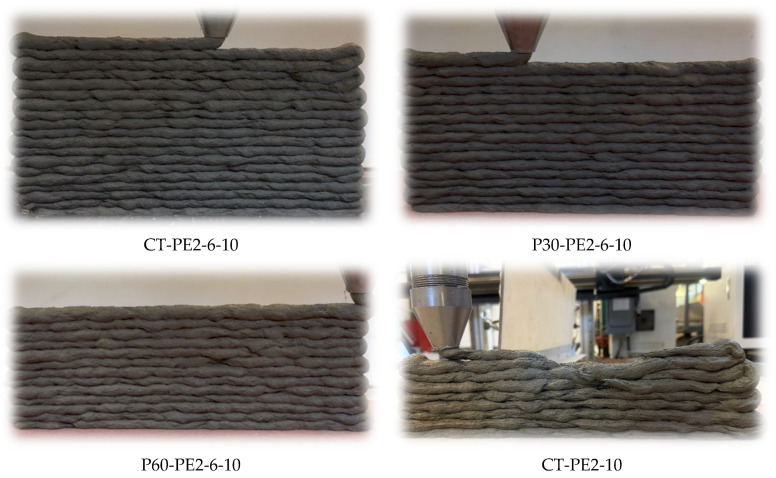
Buildability evaluation of ECC mixes CT-PE2-6-10, P30-PE2-6-10, P60-PE2-6-10, and CT-PE2-10.
